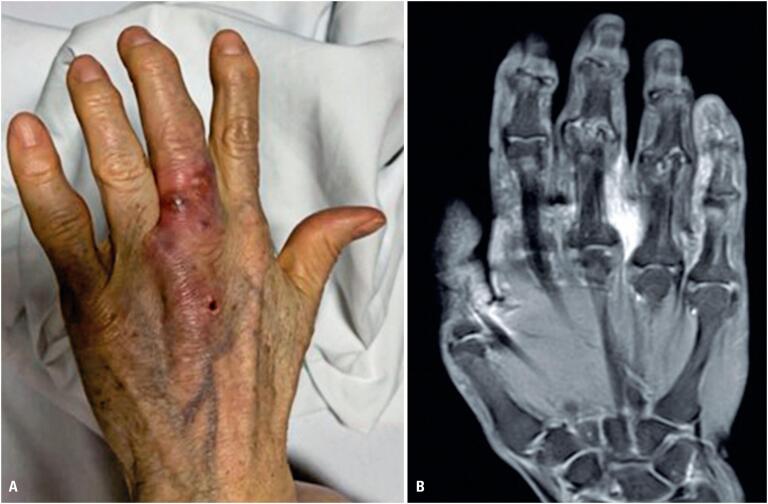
(A) Left hand injury with redness, edema, and nodulation in the third finger; (B) Magnetic resonance imaging of the left hand revealed a partially defined spindle-shaped tissue between the 3^rd^ and 4^th^ metacarpals, adjacent to the extensor tendons, involving the medial aspect of the proximal phalanx of the third finger, with adjacent liquid/edematous tissue infiltration
